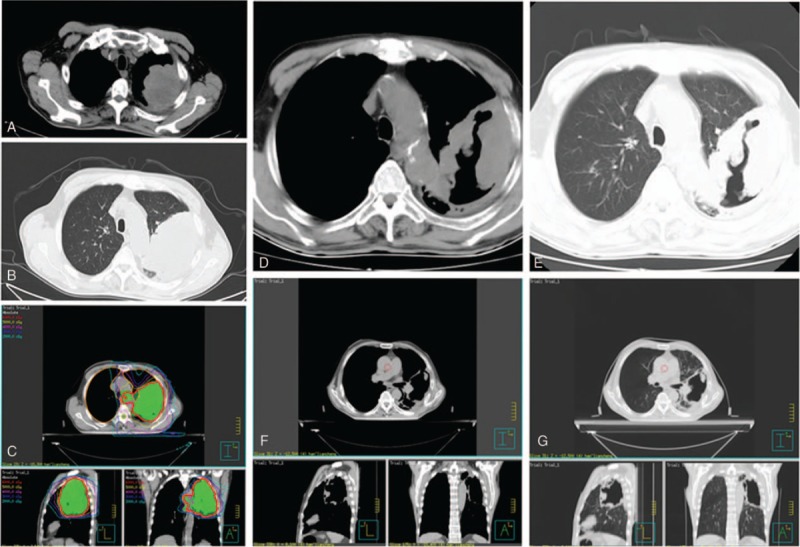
A and B: CT scan showed that there was a huge mass at the left upper lobe multiple lymph nodes metastasis in mediastinum and left hilus pulmonis. C: The target range of irradiation including the left lung primary tumor and mediastinal lymph node metastasis (V-MAT 2 Gy/F total DT: 60 Gy/30F). D and E: 1 month after treatment (January, 2017) CT scan showed that therapeutic evaluation was partial remission (PR) and the mass reduced partially. F and G: Three months after treatment (April, 2017) CT scan showed that the interior of the tumor was necrosis, cavity formation, wall nodules formation. CT = computed tomography.
